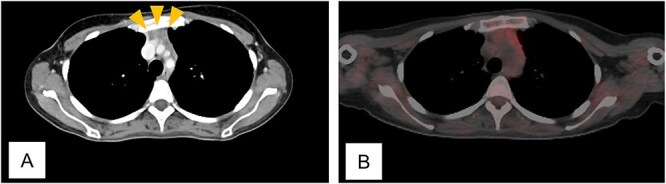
Computed tomogram and fluorodeoxyglucose positron emission tomogram/computed tomogram of Case 1. (A) Chest contrast-enhanced computed tomogram shows a 2 x 1 cm anterior mediastinal mass with increased fat attenuation (arrowhead). (B) No abnormal uptake is seen in the thymic mass on fluorodeoxyglucose positron emission tomogram/computed tomogram.
